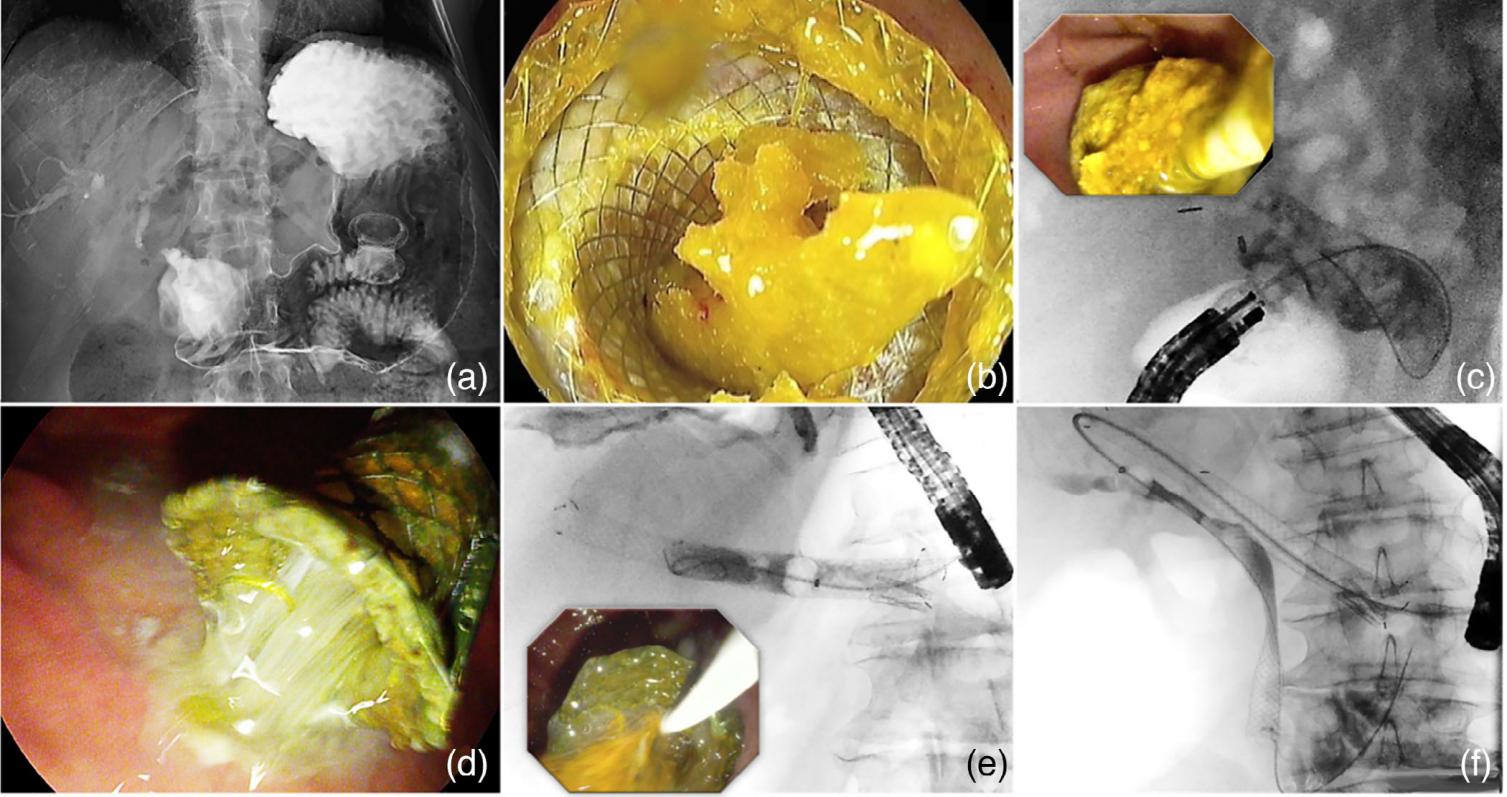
(a–c) Endoscopic ultrasound‐choledochoduodenostomy (EUS‐CDS) dysfunction. (a) During the follow‐up of a combination of EUS‐gastroenterostomy (EUS‐GE) + EUS‐CDS, the patient experienced cholangitis, and a gastrointestinal contrast series showed reflux of contrast medium inside the biliary tree through the lumen‐apposing metal stents (LAMS). (b) At endoscopic reintervention, food impaction was visible inside the choledocho‐bulbar LAMS; (c) balloon swipes were performed to extract food debris and sludge from the biliary duct. (d–f) EUS‐hepaticogastrostomy (EUS‐HGS) dysfunction. (d) During follow‐up of a combination of EUS‐GE + EUS‐HGS, the patient experienced recurrent jaundice and cholangitis. At endoscopic revision, the hepatogastric SEMS was visibly obstructed and showed an outflow of pus. (e) The SEMS was cannulated with a Fogarty balloon over the wire and balloon swipes were performed to clear accumulated debris; (f) at subsequent cholangiography, the end of the hepatogastric SEMS was angulated with respect to the direction of the left hepatic duct, and therefore biliary drainage was facilitated by anterograde placement of a transpapillary SEMS.
